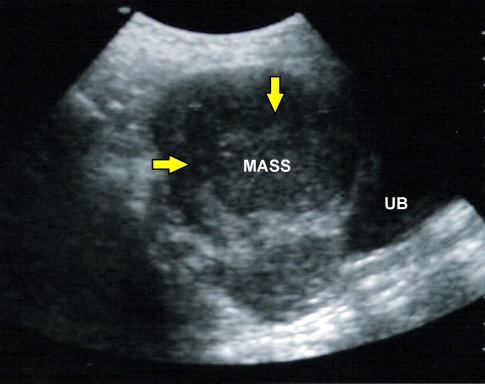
Ultrasound examination revealed a mass of mixed echogenicity in the right adnexa (arrows). UB = urinary bladder.
